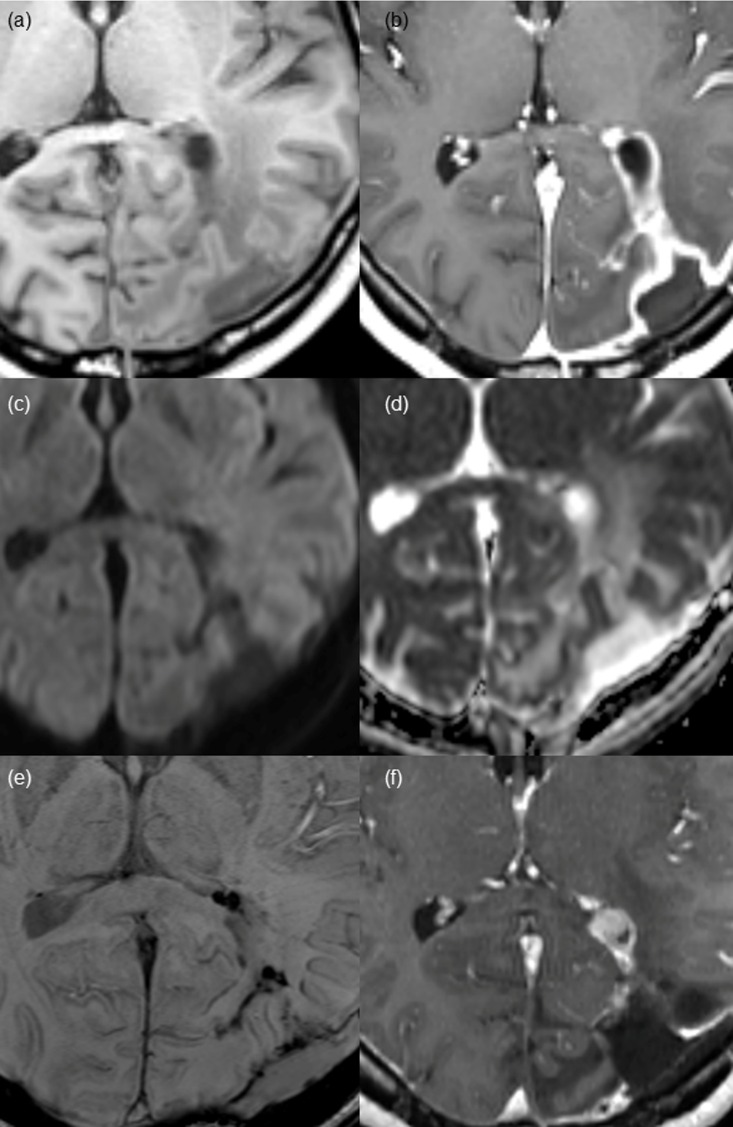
Nodular wall enhancement pattern found on post-CCRT MR imaging in a 36-year-old female patient after gross total resection of glioblastoma. (a and b) Pre- and post-contrast enhanced T1 WI demonstrates a nodular enhancement of 7 mm in the diameter at the anterior margin of the surgical cavity with thick wall enhancement. No demonstrable diffusion restrictions on (c) DWI or (d) ADC map in the enhancing portion. (e) Irregular dark signal intensity is shown along the surgical cavity on SWI, suggestive of hemorrhage. (f) At 15-month follow-up MR imaging, a measurable nodular enhancing lesion appears in the same portion of the surgical cavity, which is pathologically proven recurred glioblastoma.
